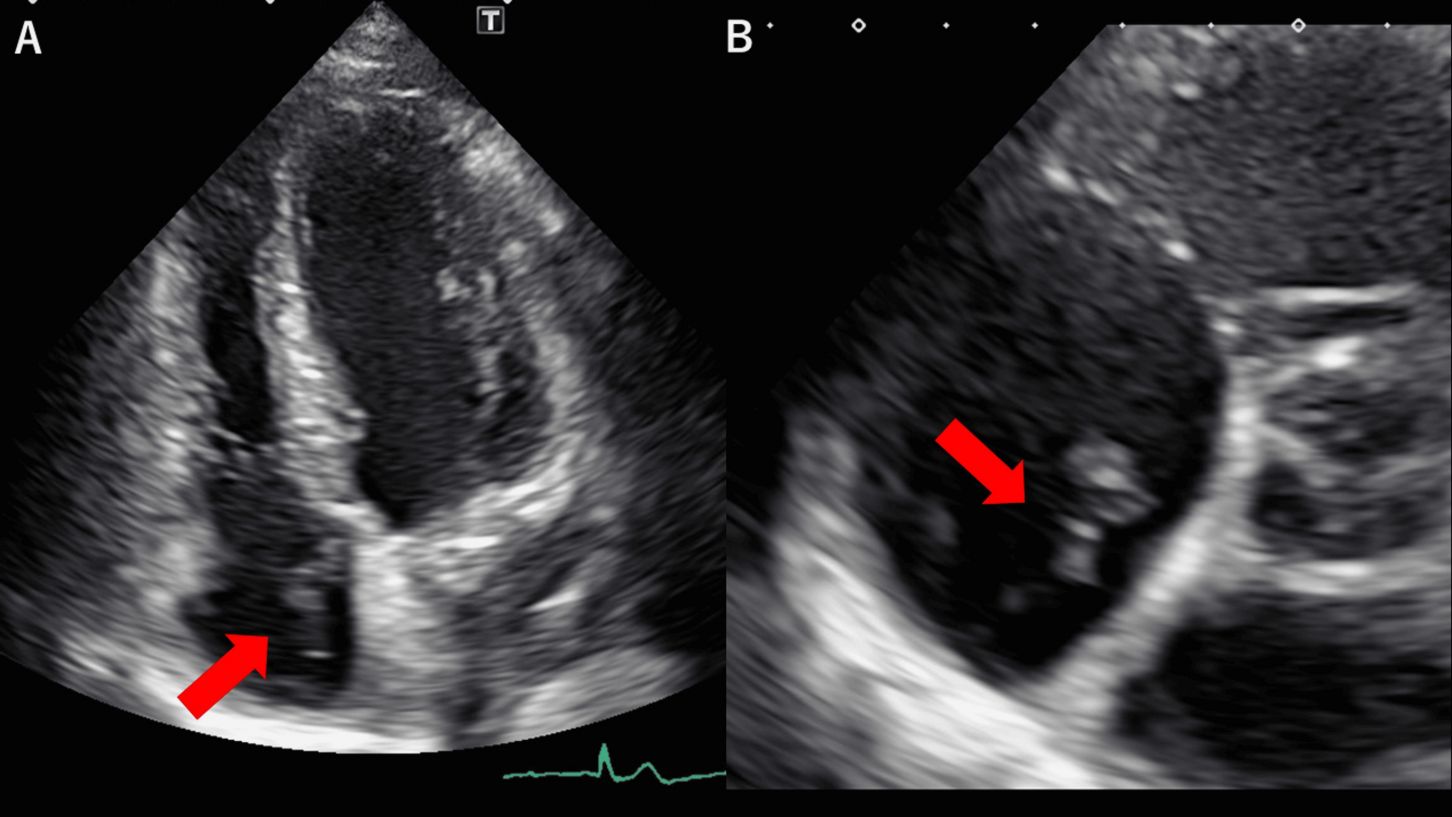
Giant Chiari's network in the right atrium Apex 4 chamber view (A) and short axis view (B) of transthoracic echocardiography. A ribbon-shaped structure is shown in the right atrium (red arrow).
